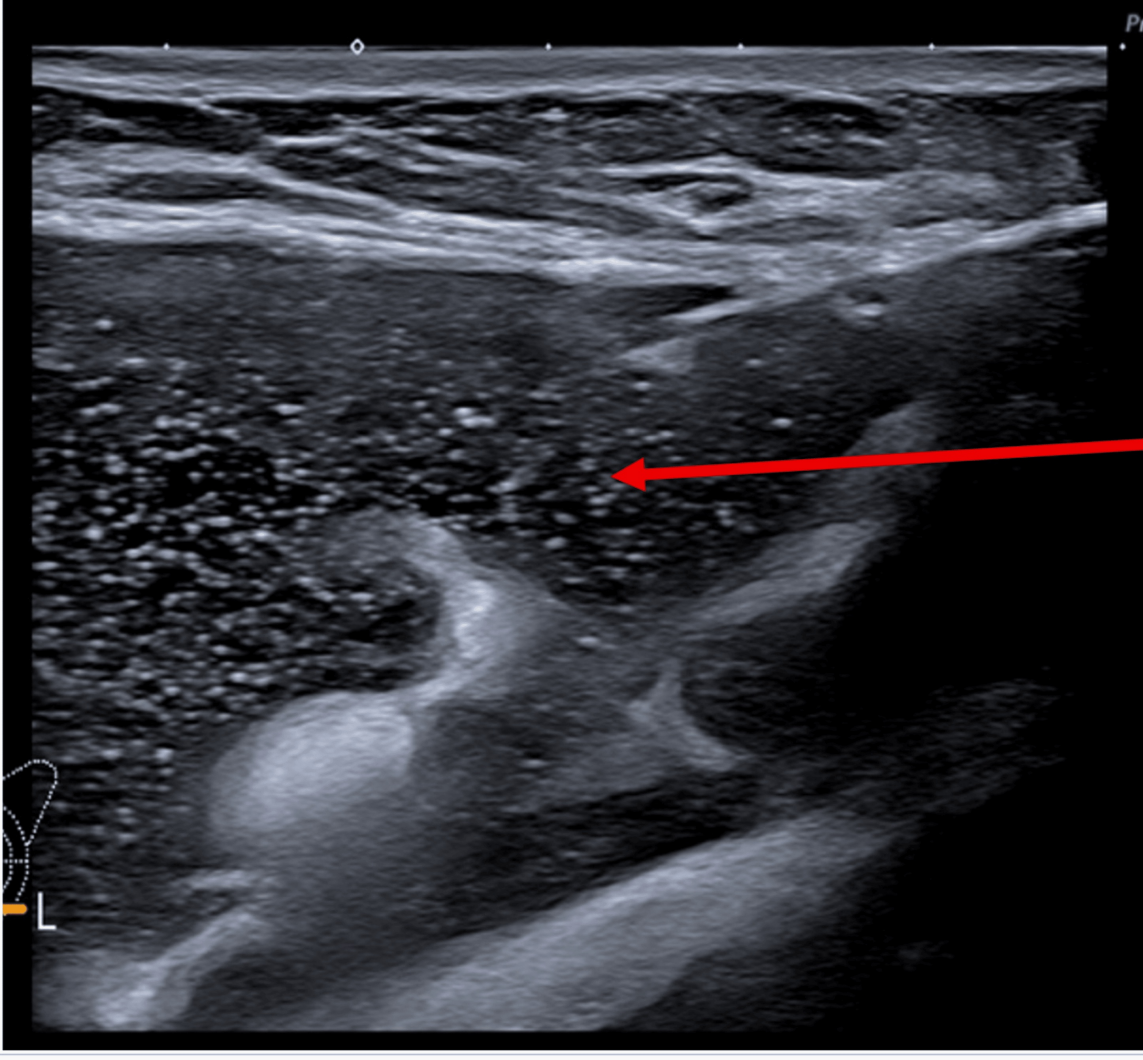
Ultrasound scan of the left breast revealing a large peri-implant collection with echogenic particles within the collection (arrow).
